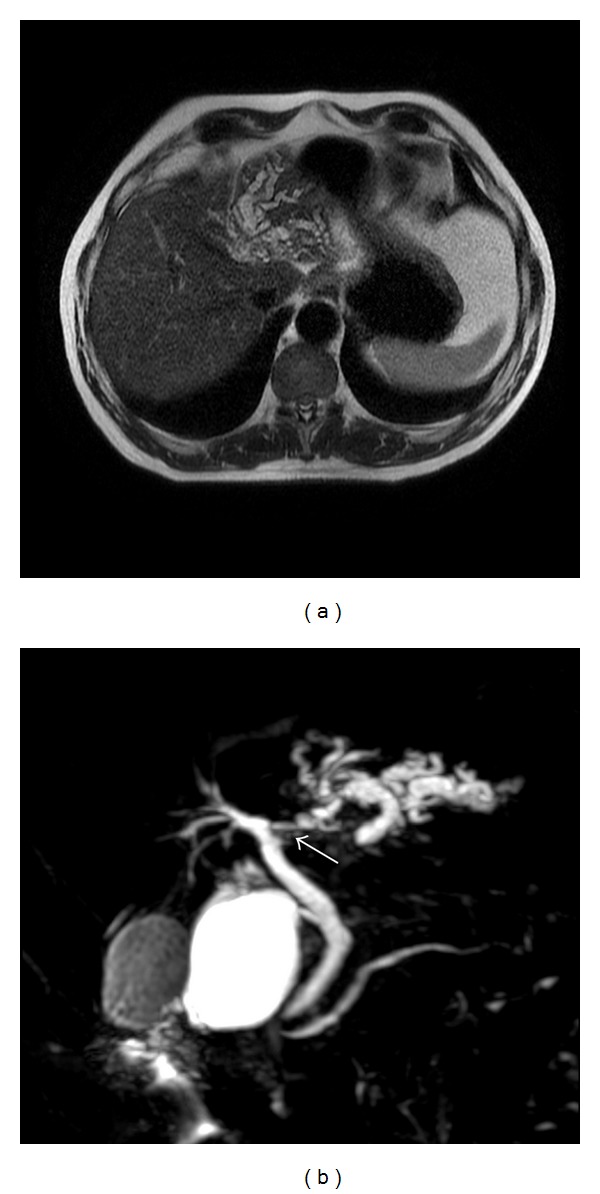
MRI demonstrates left hepatic biliary system is narrowed/occluded although a discrete mass is not well seen. MRCP demonstrates the cystic/dilated left biliary system with stricture/mass noted at the left and common bile duct junction (arrow).
